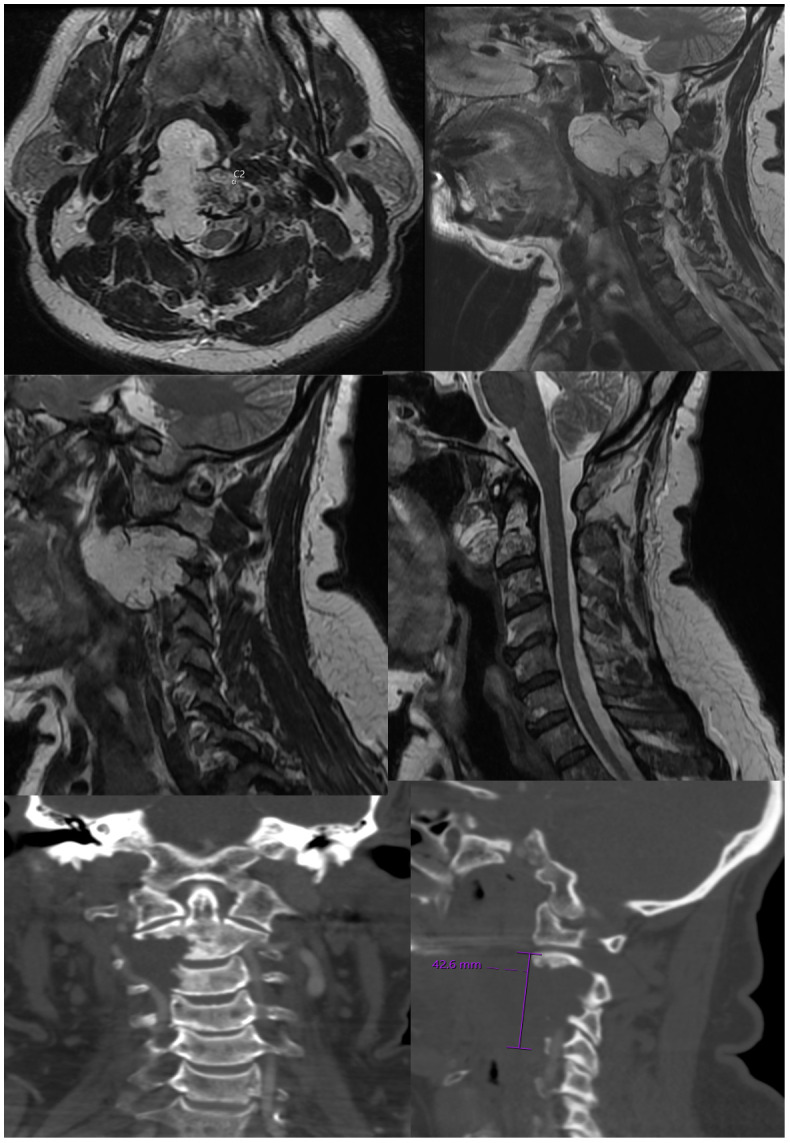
Preoperative imaging, including T2-weighted axial (upper left) and sagittal (upper right, middle images) MRI demonstrating a T2 hyperintense lesion originating from C2. CT angiogram shows encasement and displacement of the right vertebral artery (bottom left) and osseous destruction of C2 and C3 (bottom right).
